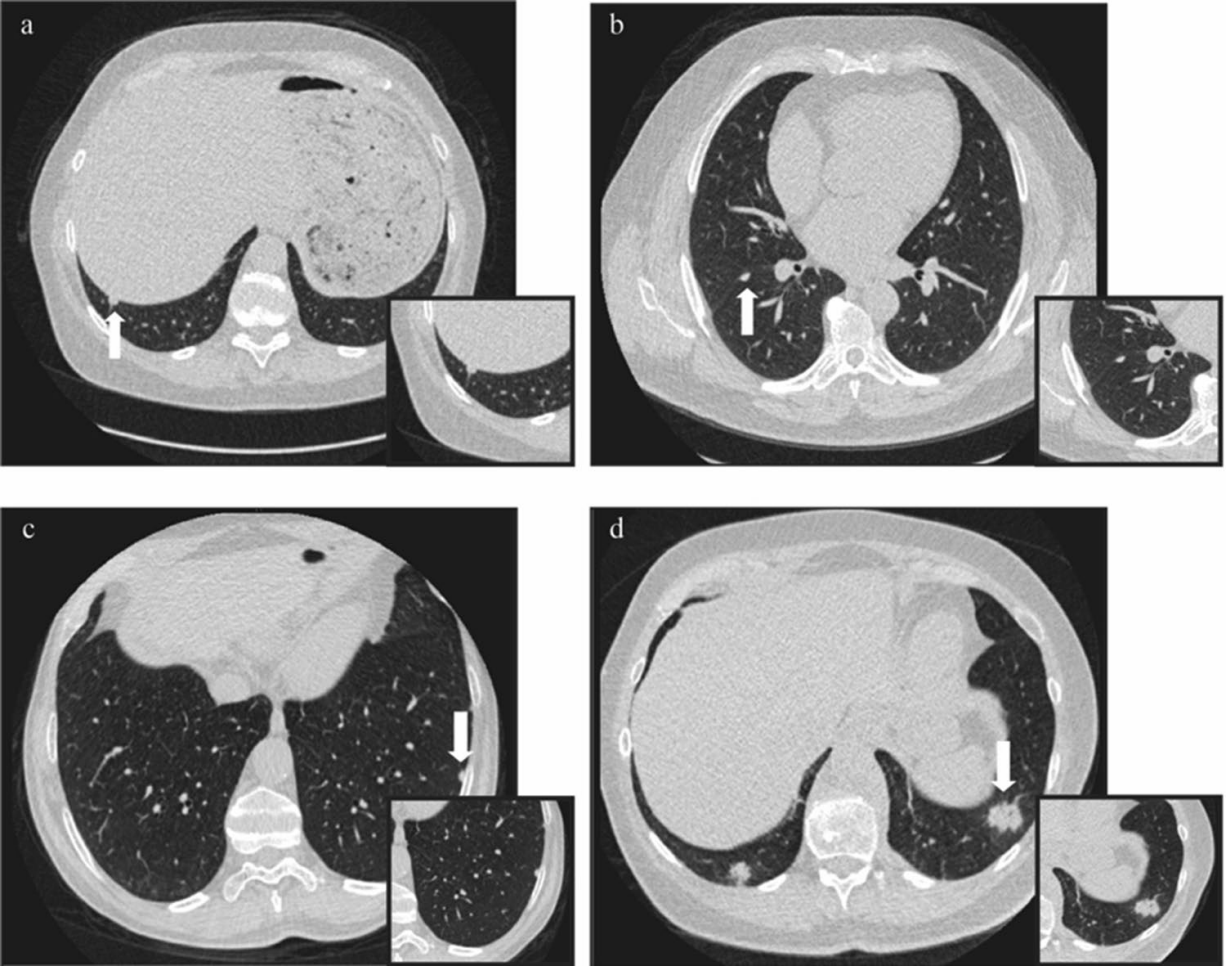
Transverse CT images of detected lung nodules in four ImaLife participants. Lung nodules indicated by white arrows, with zoomed-in view in the left bottom. (a) Solid, 136 mm^3^, oval, smooth edged, non-calcified, peripheral lung nodule, in the right lower lobe posterior basal segment. (b) Solid, 132 mm^3^, triangular, smooth edged, non-calcified, peripheral lung nodule which was categorised as a typical perifissural nodule (PFN), in the right lower lobe apical segment. (c) Solid, 154 mm^3^, triangular, smooth edged, non-calcified, peripheral lung nodule which was categorised as an atypical PFN, in the left lower lobe lateral basal segment. (d) Solid, 3933 mm^3^, irregular shaped, irregular edged, non-calcified peripheral nodule, situated in the left lower lobe anterior segment.
